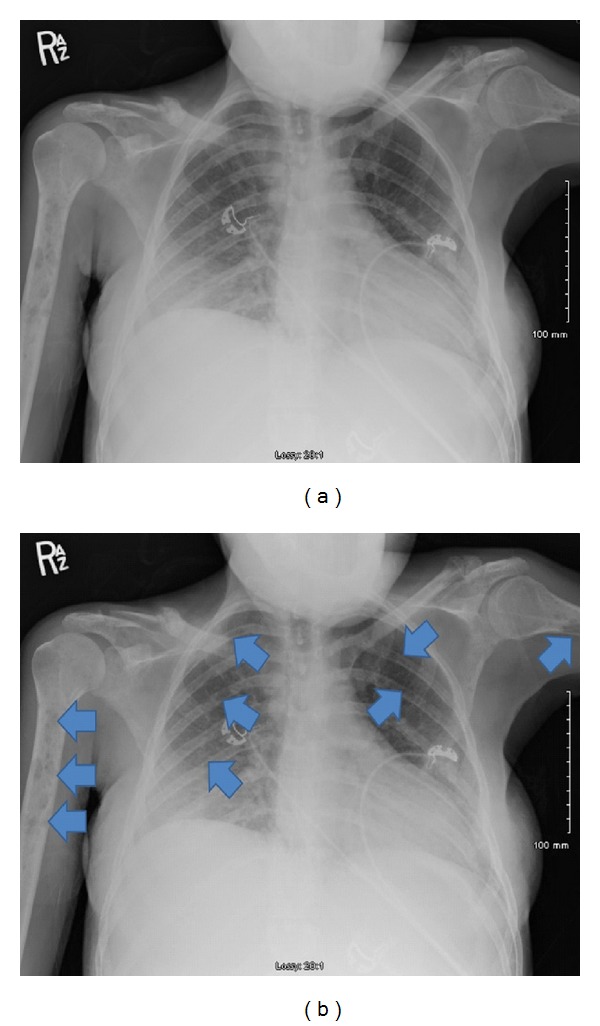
Chest radiograph of patient showing diffuse lytic bone lesions (indicated by blue arrows), most prominent in bilateral humeri and healed fracture deformities in the clavicles and ribs.
